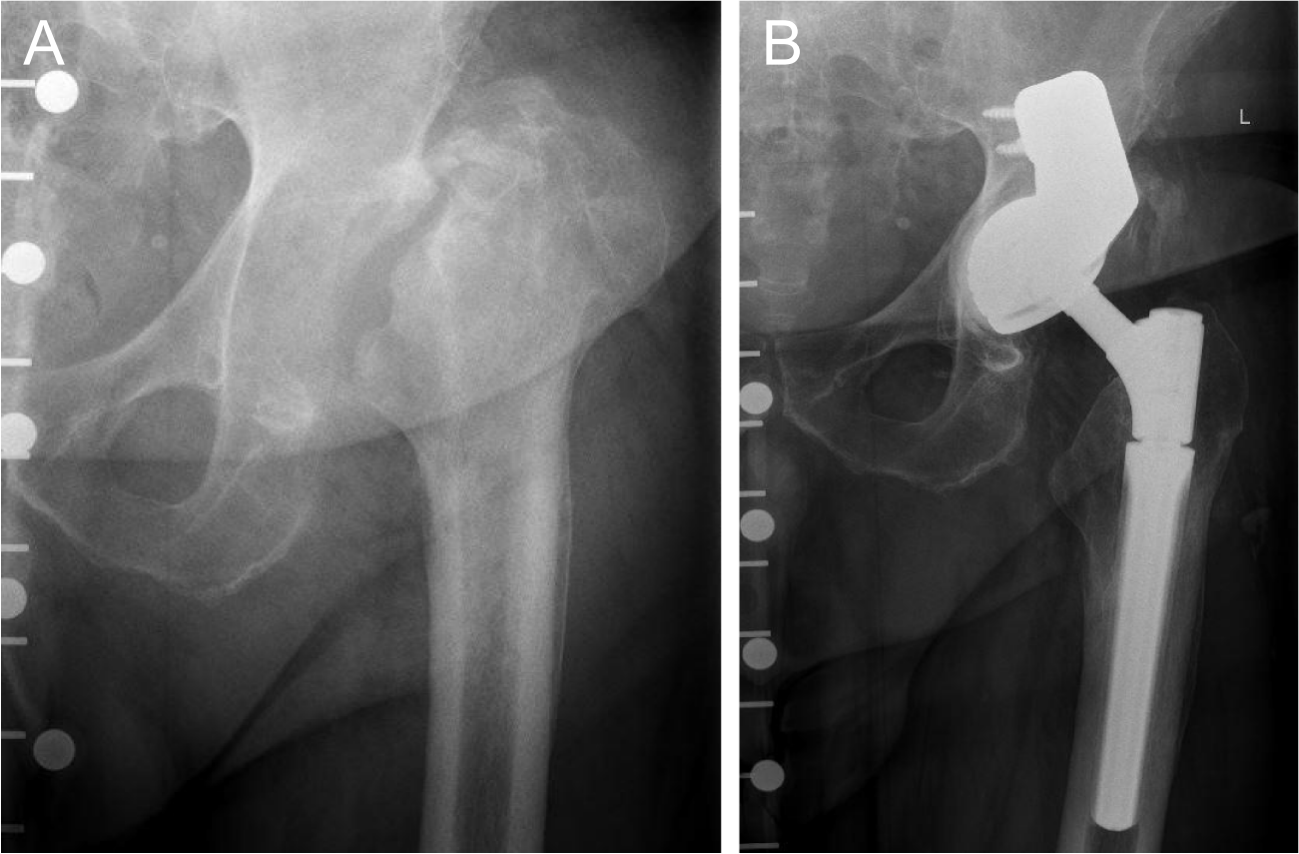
A Preoperative anteroposterior radiograph of the hip of a 56-year-old patient with severe acetabular bone loss including severe posterior acetabular rim defect due to infection and two-stage revision THA. B Anteroposterior radiograph of the same patient 4.5 years after surgery using a modular tantalum TM buttress augment and a cemented dual mobility cup. There are no signs of loosening of one of the components
